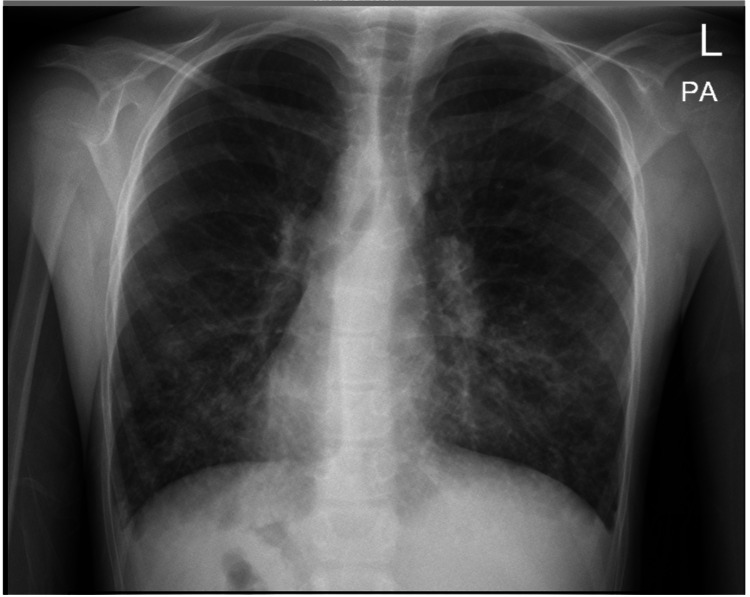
Chest x-ray (posterior anterior view) showing dextrocardia
